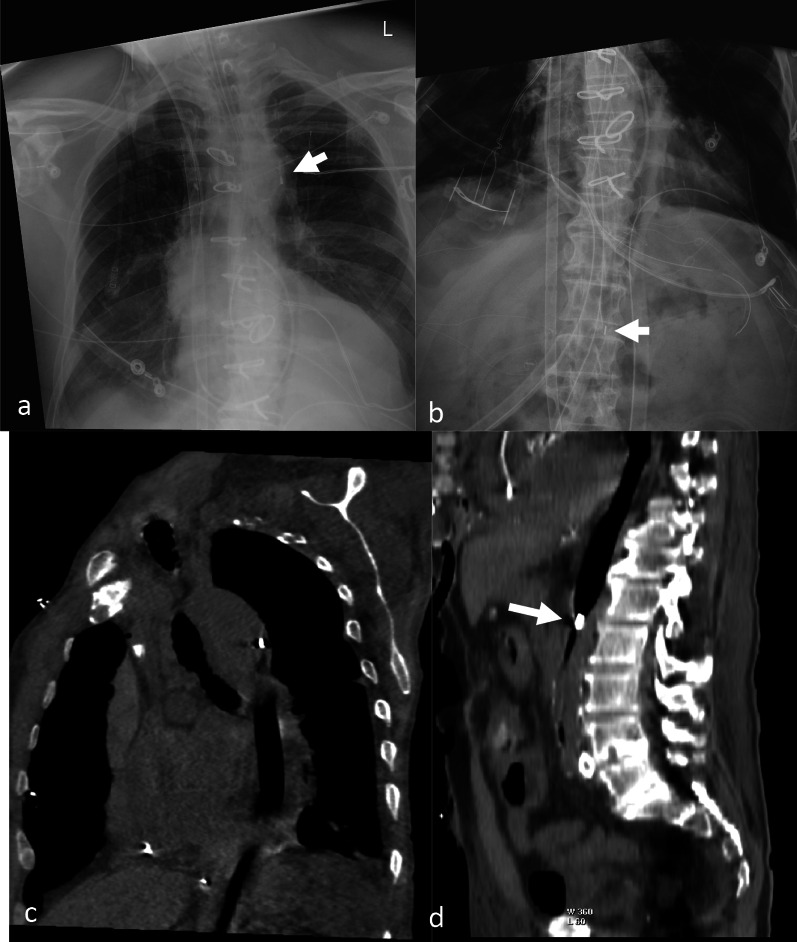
Intra-aortic balloon pump (IABP). Chest AP radiograph (a) demonstrating the upper radiopaque marker (white arrow) projecting over the aortic knob superior to the carina. This is the expected position based on radiography. Abdomen AP radiograph (b) in the same patient demonstrates the IAPB with the inferior radiopaque marker (white arrow) projecting over the L1-L2 Intervertebral space, which is low. Oblique coronal CT (c) demonstrates the IAPB in the descending aorta. The radiopaque marker is visible within the descending aorta about 5 cm from the origin of left subclavian artery. Sagittal CT abdomen image (d) demonstrates the low position of the IABP with its inferior radiopaque marker (black marker) below the level of the diaphragm at the level of the L1–L2 Intervertebral space. This can be seen when there is anatomic-to-balloon length mismatch
